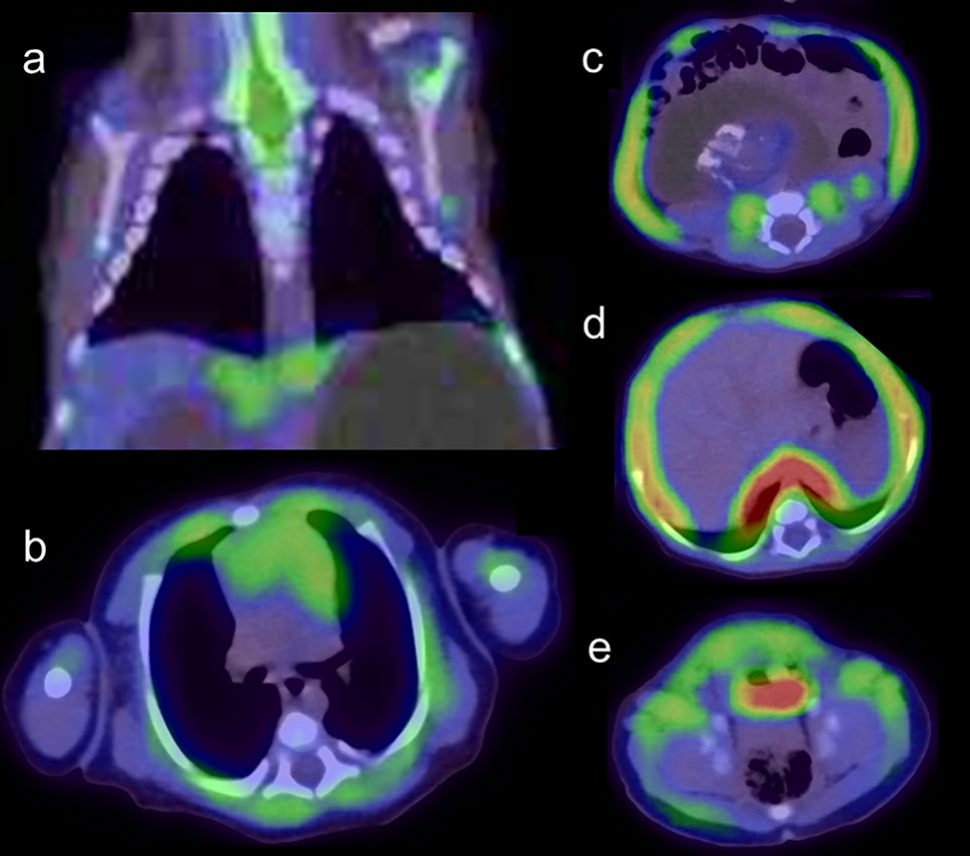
FDG PET/CT image of neonates with15 days after birth. a the neonatal diaphragm is flatter and attaches to the chest wall at a wider angle. b physiological FDG uptake in thymus, and intercostal muscles supporting breathing. a–e abdominal muscle supporting breathing and diaphragm
